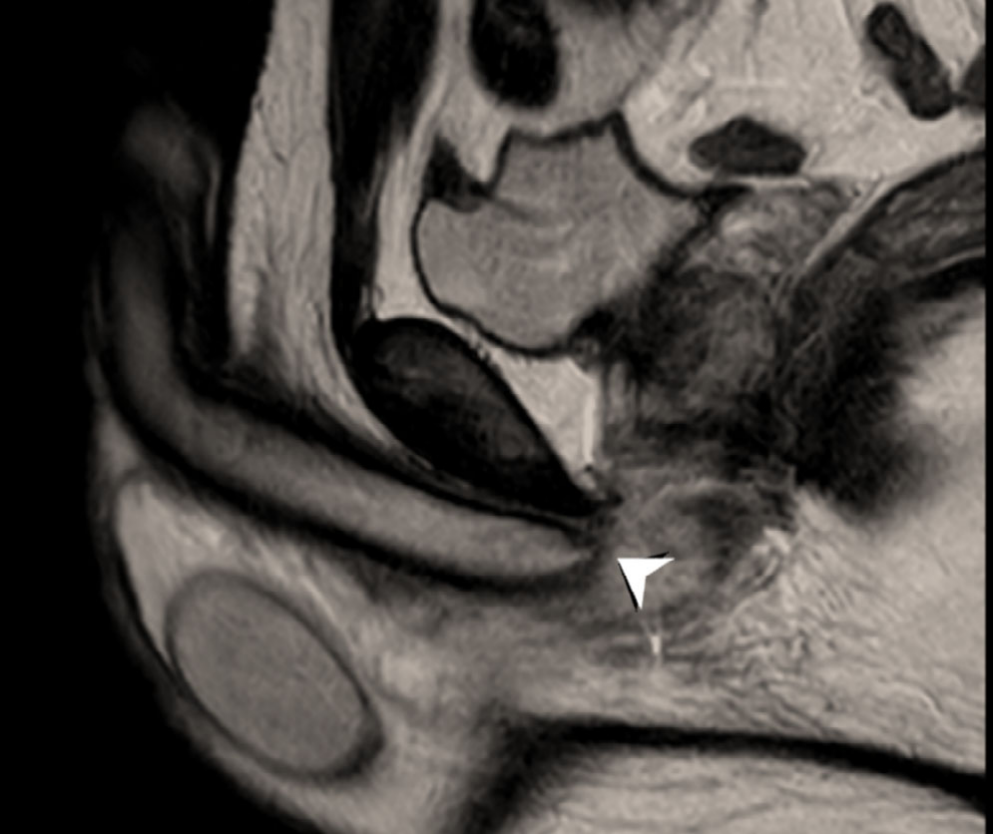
Sagittal T2‐weighted MRI image of the penis shows a small beak‐like protrusion (white arrowhead) near the base of the right corpus cavernosum in keeping with a small tear.
